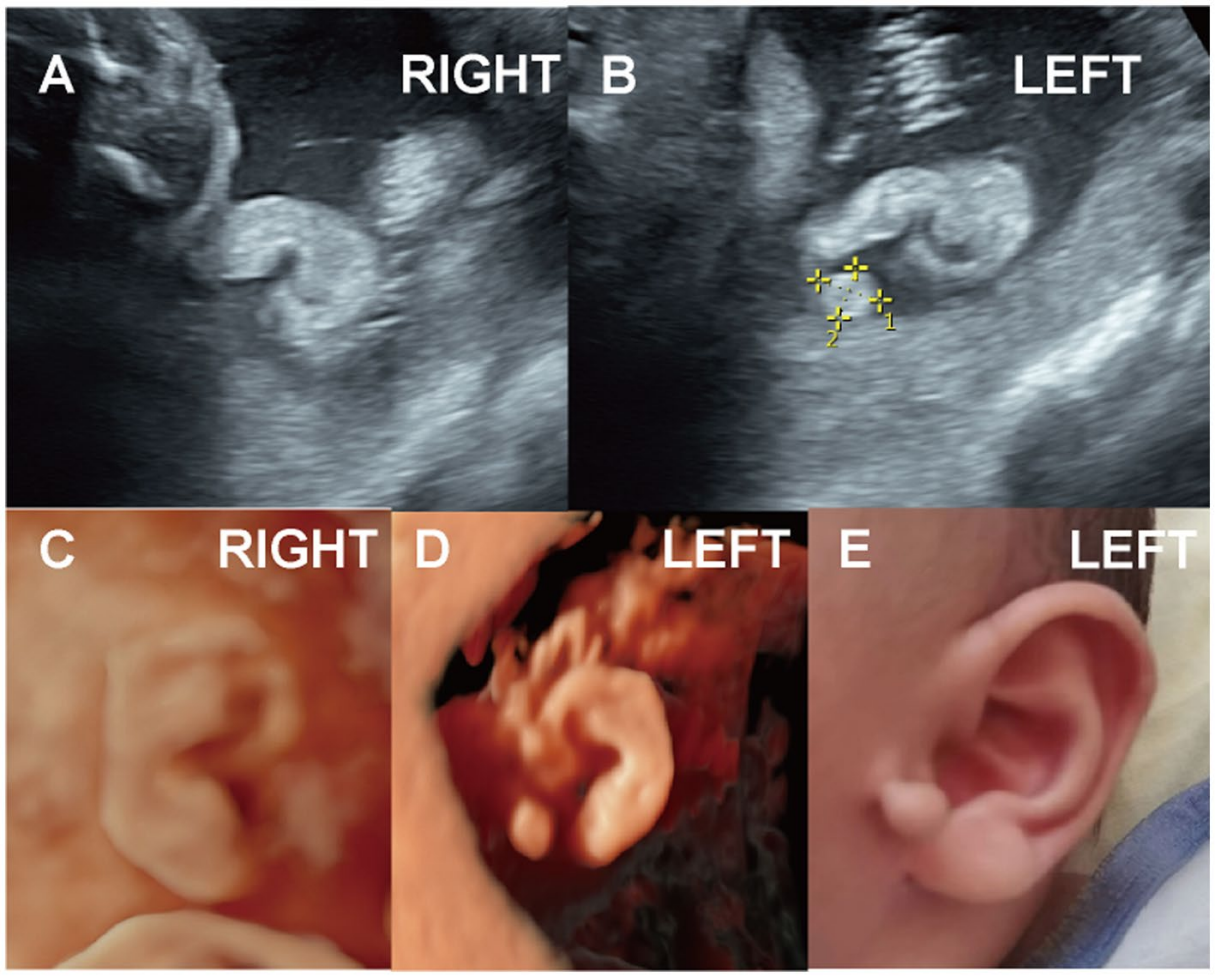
(Case 11). A pregnant woman, whose husband had an accessory auricle on his right ear, underwent ultrasound at 22 weeks 6 days. (A-D) The scan revealed a moderately echogenic accessory auricle on the left ear of the fetus, measuring 0.8 × 0.6 cm. No other abnormalities were noted, and genetic testing was not performed. (E) A full-term baby boy was delivered with an accessory auricle located below the left earlobe. Hearing test results were normal.
